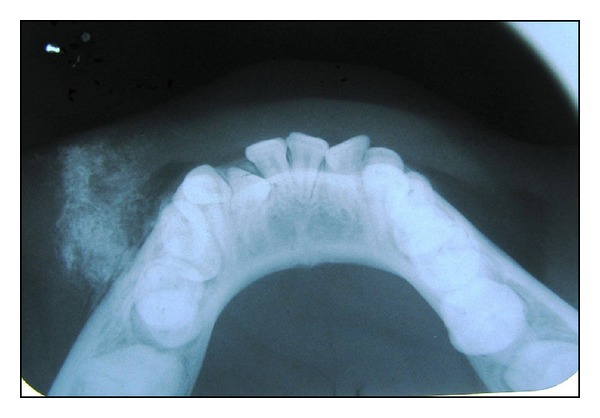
Preoperative occlusal radiograph revealed irregular radiopacities extending into soft tissue shadow of buccal mucosa and lower lip from the buccal cortex.
